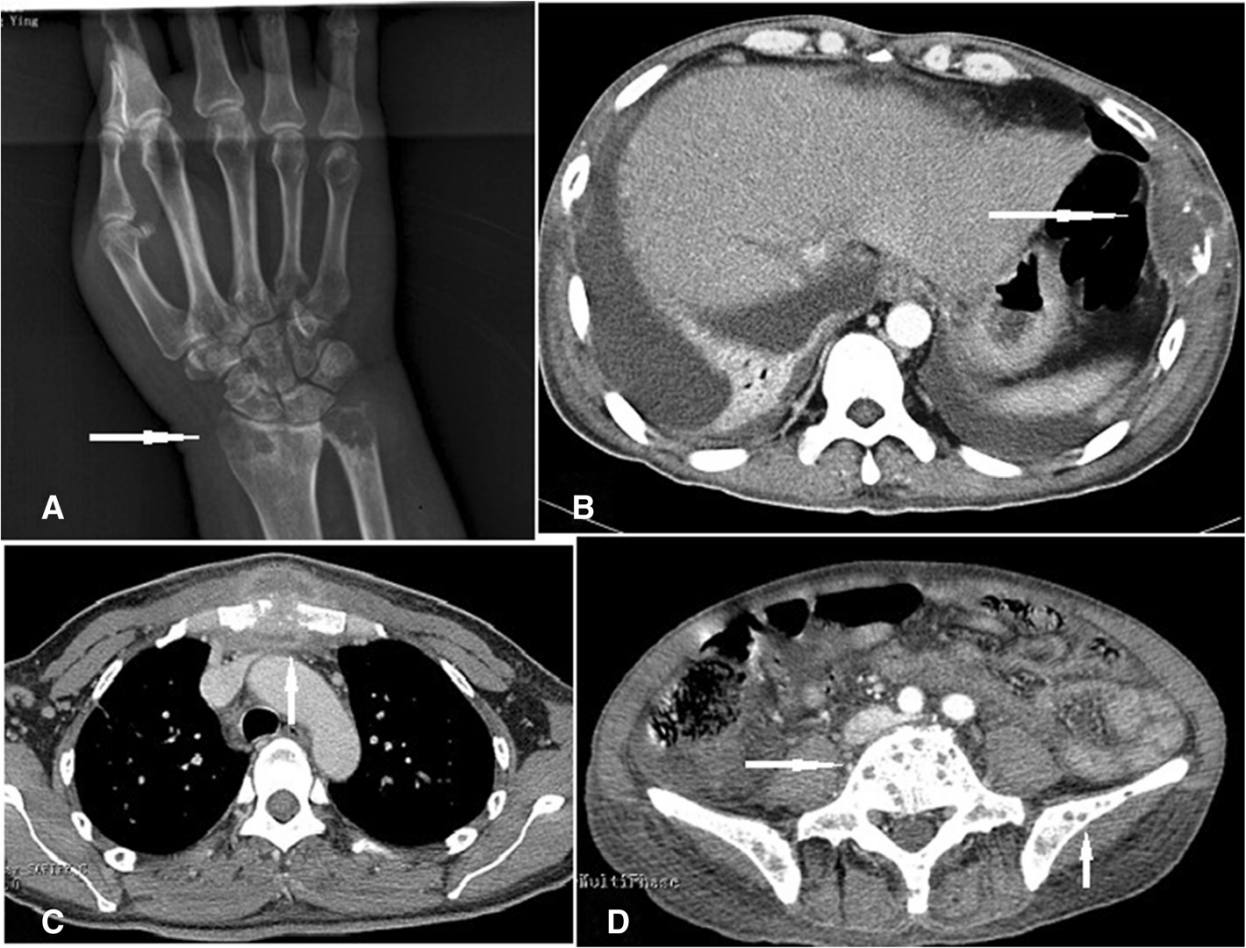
Radiography and computed tomography showing osteolytic lesions characterized by multiple lucent defects with a moth-eaten destruction of bone in the head of the radius (A), ulna (A), rib (B), sternum (C), vertebrae (D), and ilium (D), and swelling in surrounding soft tissues (B, C).
